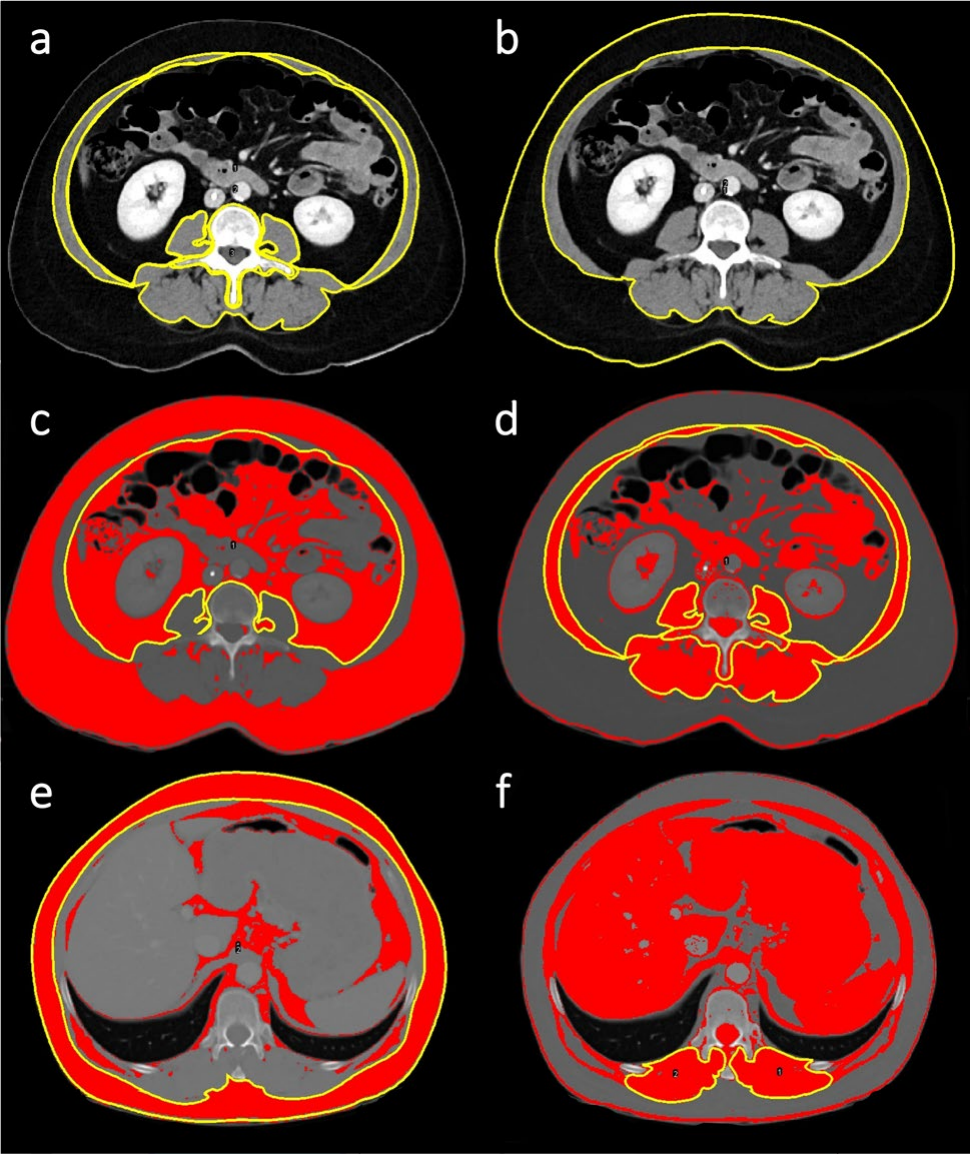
Regions of interest (ROI) for fat and muscle measurements on axial CT images. Measurements were performed on axial slices of contrast-enhanced CT scans of the thorax and abdomen at the level of the third lumbar vertebra (L3) (a–d) and the twelfth thoracic vertebra (T12) (e–f) with a muscle-specific (− 29 to + 150 Hounsfield units (HU)) and fat-specific threshold (− 190 to − 30 HU), respectively. Image (a) and (b) are pictured without the threshold to allow better visibility of anatomic structures. For the skeletal muscle area (SMA) at L3, the area of the ROI around L3 and of that around the abdominal muscles' inner circumference were subtracted from the area of the ROI along the outer muscles' circumference according to Gomez-Perez et al.^25^ (a). For the subcutaneous fat area at L3 and T12 the area of the ROI along the outer muscles' circumference was subtracted from the area of the ROI around the waist circumference (b, e). For the visceral fat area, a ROI was drawn along the inner circumference of the abdominal muscle (c). The circled muscles used to determine the muscle radiodensity attenuation (MRA) at L3 are shown in (d). For the SMA and MRA at T12, because of a potential measurement bias caused by the ribs, ROIs were defined along the posterior paraspinal muscles' circumference (f).
